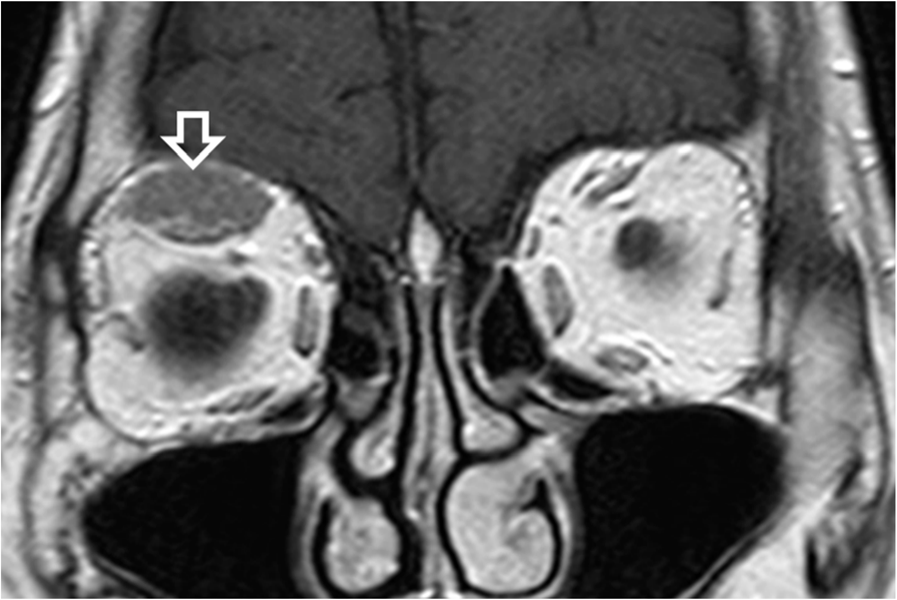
Magnetic resonance imaging of an 82-year-old man with right orbital MALToma presenting as painless orbital swelling. Coronal T1-weighted post-contrast image shows a homogeneous moderately contrast-enhancing MALToma in the right superior rectus muscle (open arrow), a common site of orbital MALToma
